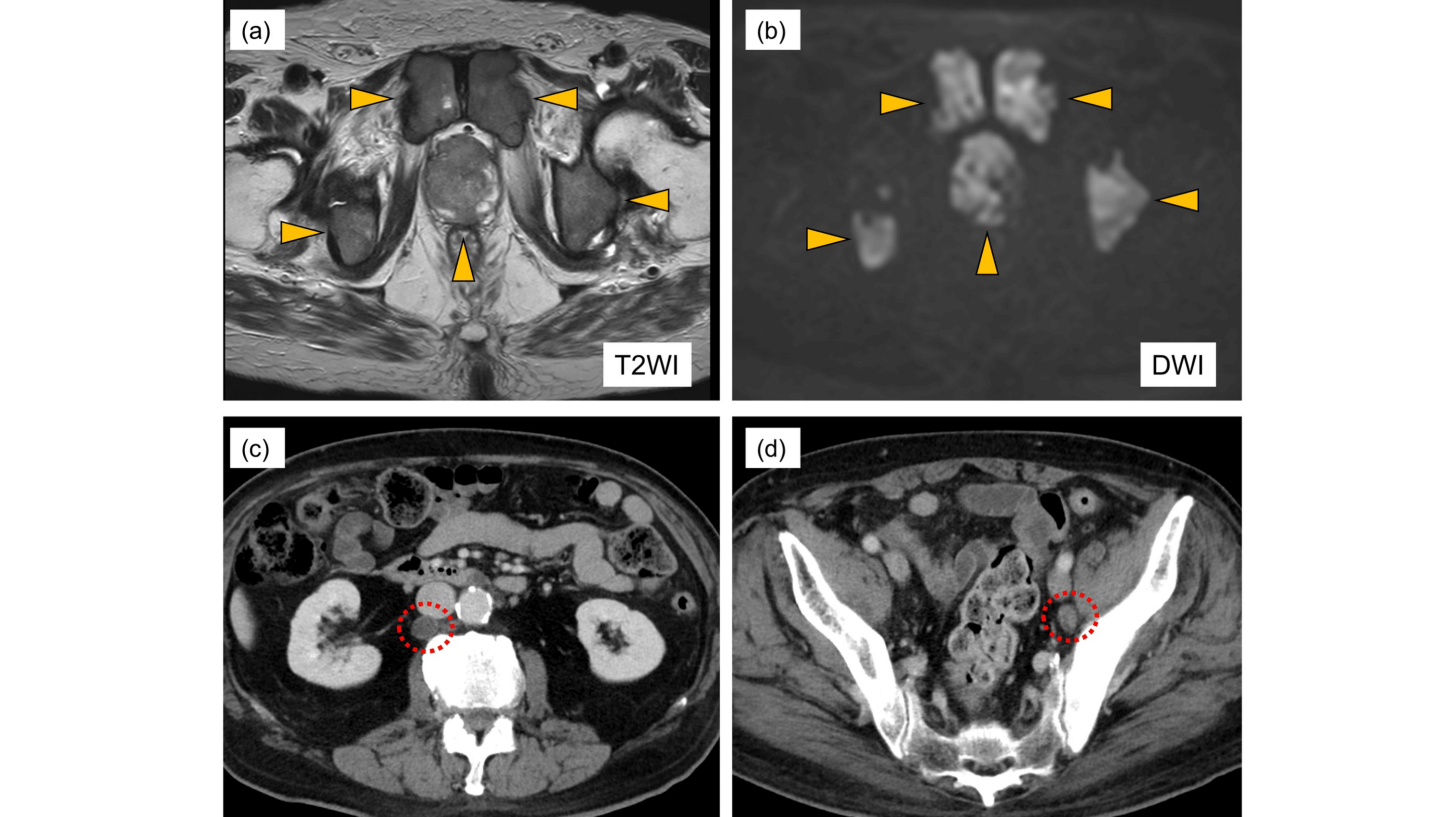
MRI and contrast-enhanced CT images. (a, b) MRI shows an extensive prostate tumor with multiple pelvic bone metastases. The arrows indicate the bone metastasis. (c) Contrast-enhanced CT demonstrates enlargement of para-aortic lymph nodes. (d) Contrast-enhanced CT reveals enlargement of the left internal iliac lymph nodes. The circles indicate the lymph node metastasis.
